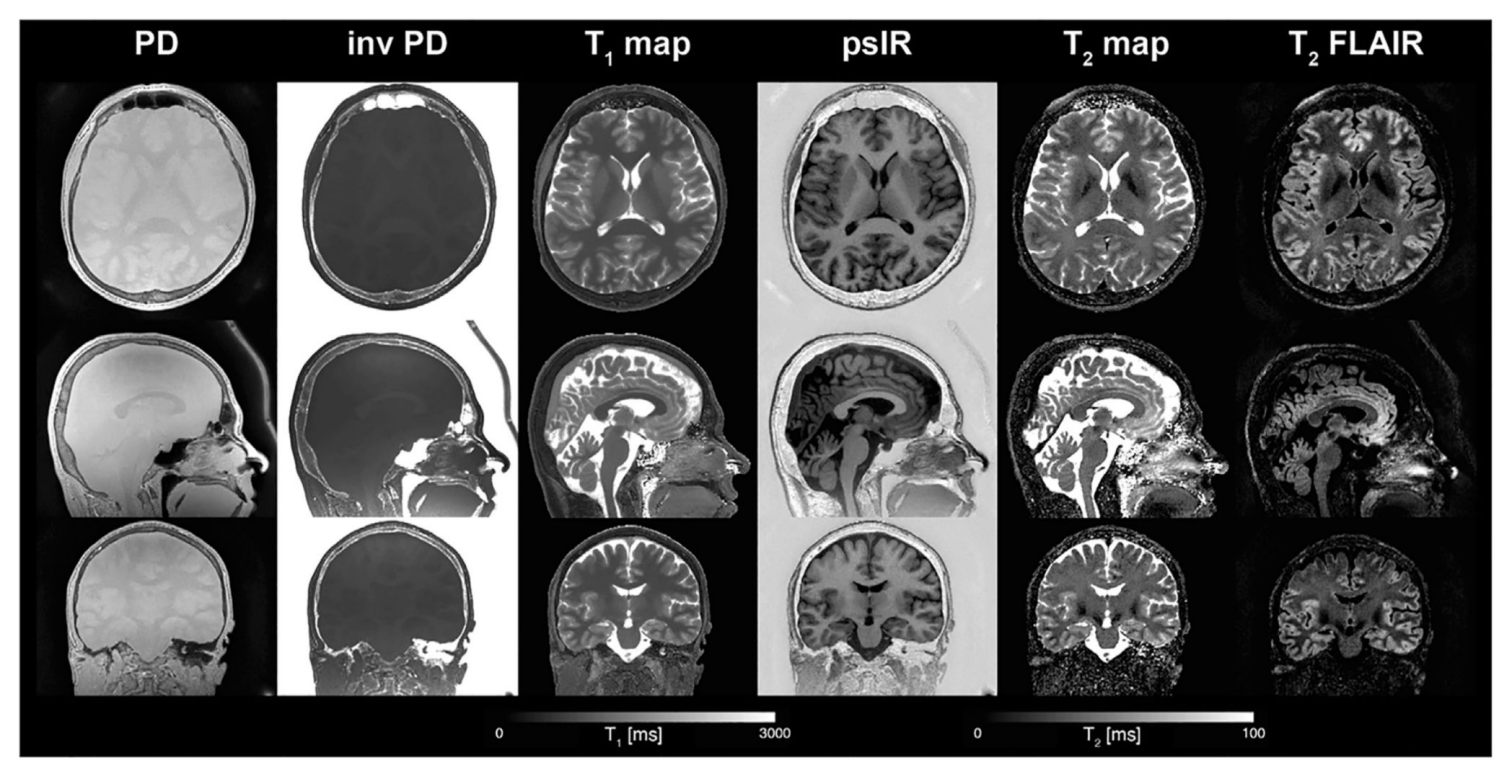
Quantitative PD, T_1_ and T_2_ maps obtained using a multi-parametric ZTE sequence together with synthetic contrast weighted psIR and T_2_ FLAIR images. Acquisition parameters: FOV = 20 × 20 × 16 cm^3^, resolution = 1 × 1 × 1 mm^3^, TR = 1.8 ms, BW_RX_ = ±31.25 kHz, TE _T2Prep_=80 ms, 256 spokes per segment. FA = 3° for multi-parametric part and FA = 1° for PD volume. Total acquisition time was 6:35 min. The T_1_ map and T_2_ map have been head masked using the PD volume. Abbreviations: inv PD – inverse PD, psIR – phase sensitive inversion recovery, FLAIR – fluid attenuated inversion recovery. Images have been cropped to head coverage. Fig. 10 shows the PD data reconstructed at twice the prescribed field of view. (Images generated with data from Ref [114]).
